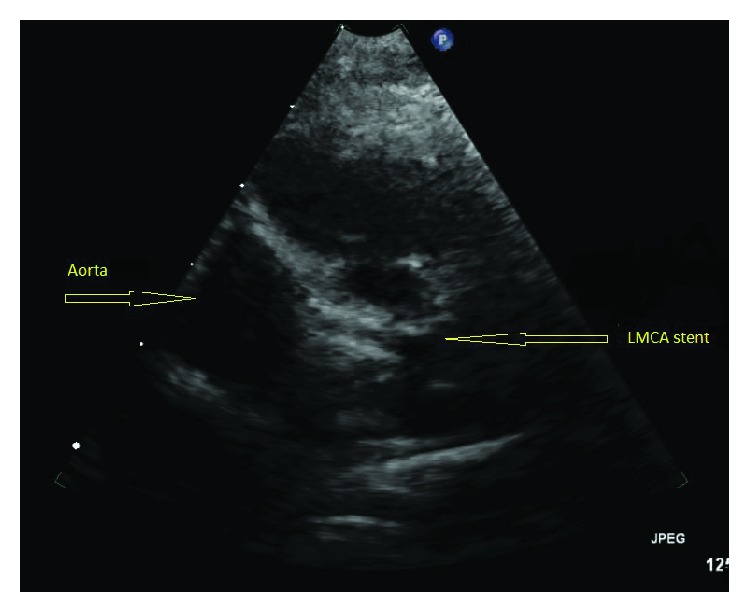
Post-PCI transthoracic echocardiogram demonstrating widely patent stent in the LMCA.
